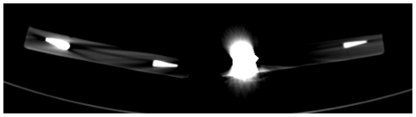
Streak artifact produced by Zirconium dioxide in CT.
